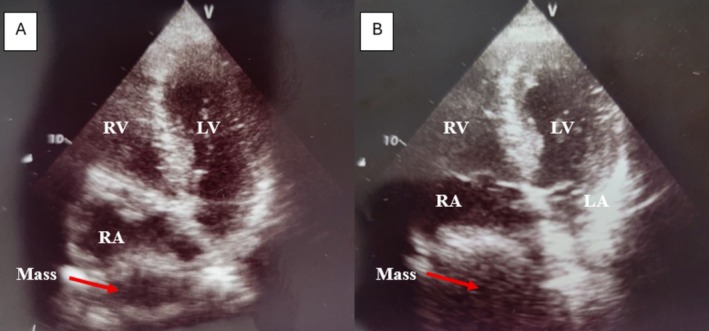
Mass encroaching on the right atrium on the four‐chamber apical view on 2‐D transthoracic echocardiography. RV = right ventricle; LV = left ventricle; RA = right atrium; LA = left atrium; Mass (Arrow).
